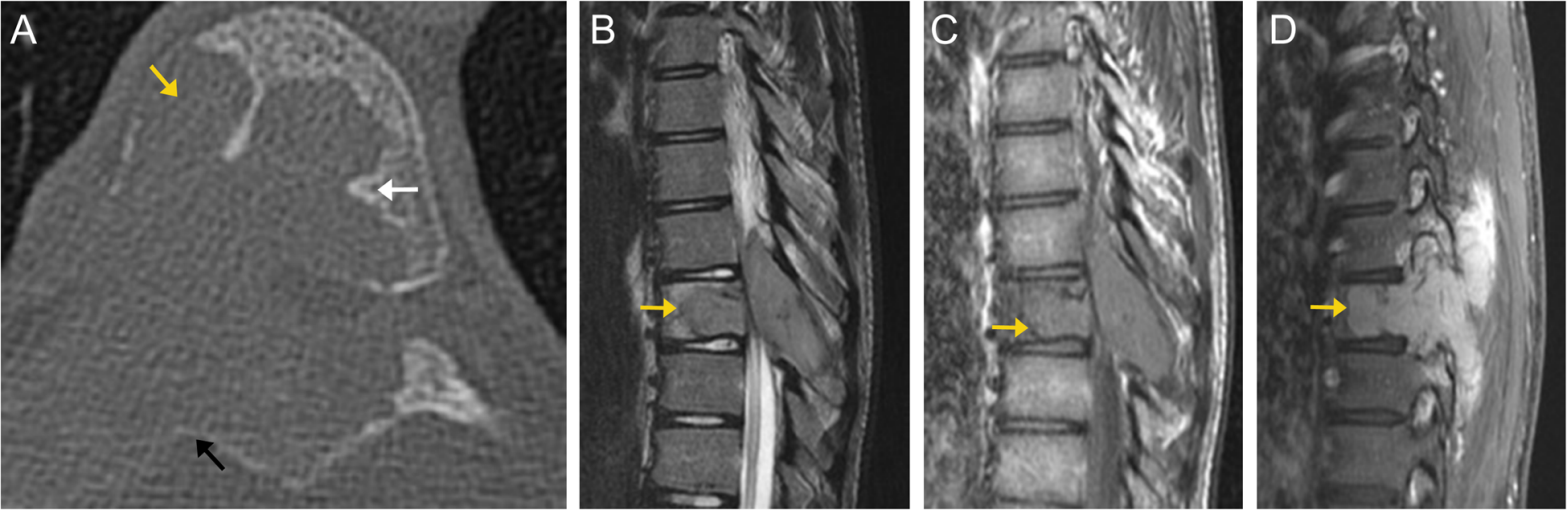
Osteoblastoma. Axial (a) CT image of the thoracic spine demonstrates a lytic lesion involving the T7 vertebral body (orange arrow) and posterior elements, with an expansile soft tissue component. A thin sclerotic rim can be seen along the border of the intervertebral portion of the lesion (white and black arrows). Sagittal T2 (b), T1 pre-contrast (c), and T1 post-contrast (d) MR images again demonstrate a lesion involving the T7 vertebral body and posterior elements (orange arrows), as well as invasion of the adjacent paraspinal soft tissues. The lesion is predominantly low to intermediate signal compared to normal marrow, and demonstrates avid enhancement on post-contrast images
